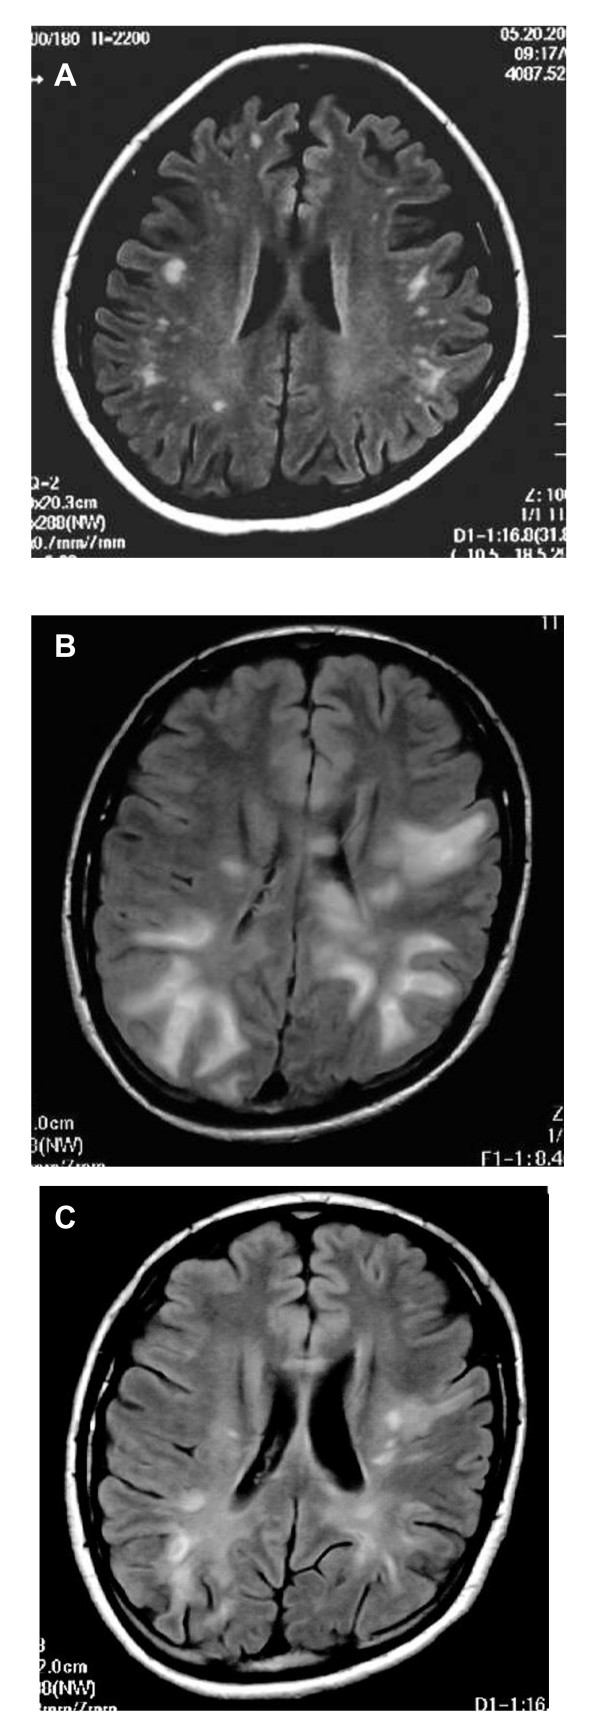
Abnormal magnetic resonance imaging signals in patients with systemic lupus erythematosus (SLE). (A) Typical white-matter lesions in central nervous system (CNS) lupus in a fluid-attenuated inversion-recovery (FLAIR) image of a 21-year-old woman having a headache caused by benign intracranial hypertension. Many foci of small-sized abnormal signals are visible in the white-matter of the frontal and parietal lobes. This type of abnormality was also observed in some of the SLE patients who had no history of CNS syndrome. (B) FLAIR image of a 23-year-old woman with demyelinating syndrome and acute confusional state, showing multiple large hyperintensities involving both grey and white matter (Case 3 in Table 5). (C) Remarkable resolution of the clinical signs and imaging picture 3 months later, following high dose glucocorticoid with intravenous cyclophosphamide therapy.
